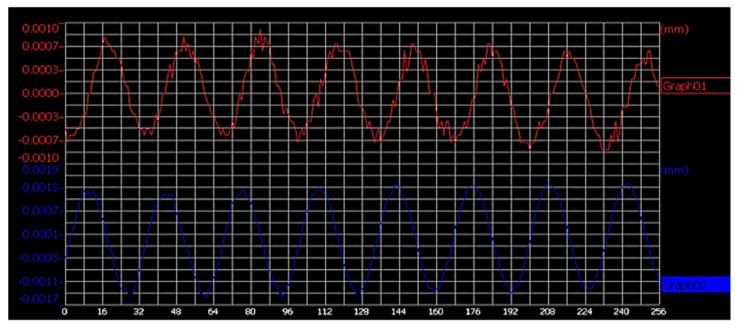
Ultrasonic amplitude test results.
